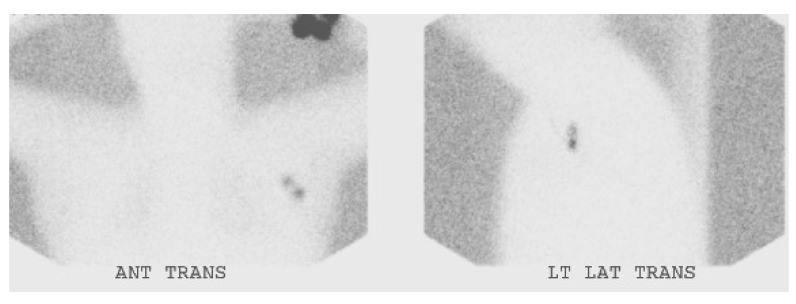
Sentinel node scintigraphy in a patient with MCC of the left forearm.
